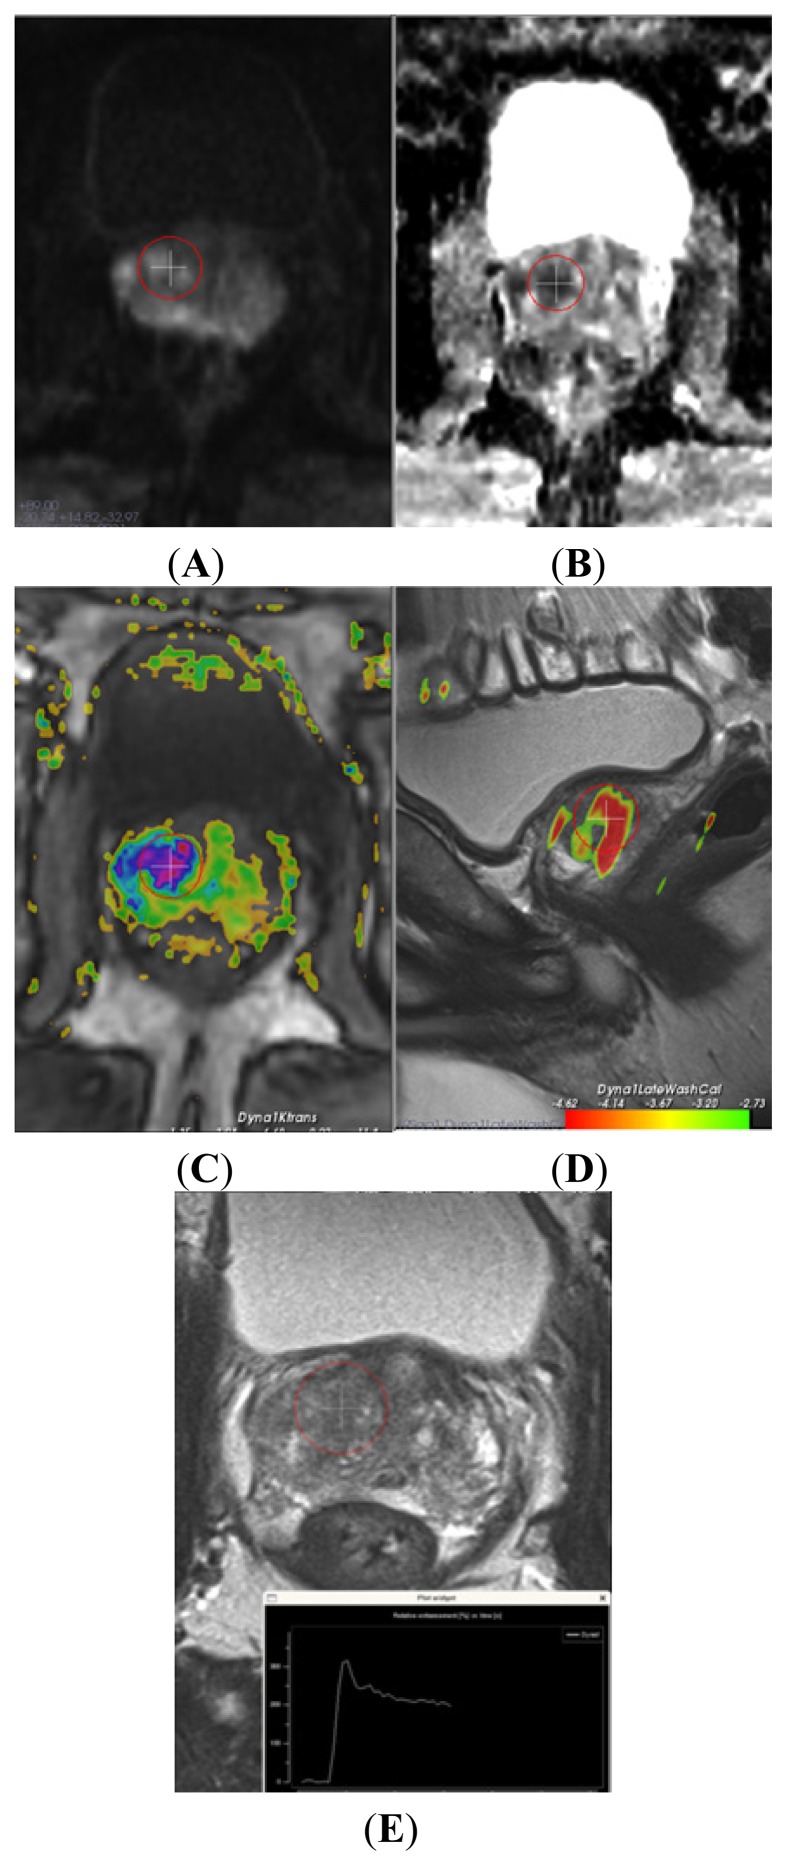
Multiparametric magnetic resonance (MR) imaging of the prostate of a 77 year old male, PSA level of 53, prostate cancer gene 3 (PCA3) score of 43 and 2× negative transrectal ultrasound-guided biopsy sessions. (A) axial diffusion weight imaging (DWI-b 1400) shows a high signal; this combined with a low signal on the (B) axial apparent diffusion coefficient (ADC) map, indicating restriction and, thus, suspicion for intermediate grade cancer. (C) axial and (D) sagittal dynamic contrast-enhanced MR images show increased vascular permeability. The curve (bottom) has a steep rise and wash out, which indicates cancer; (E) axial T2 weighted MR image shows a homogeneous low signal, which also fits tumor. MR-guided biopsy revealed in all three cores 75% Gleason 4+3 prostate cancer.
